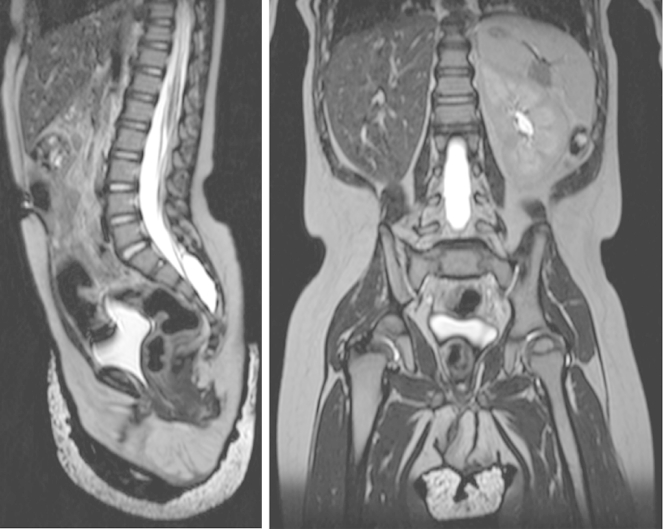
Sagittal (left) and coronal (right) T_2_-weighted magnetic resonance imaging views of the abdomen and pelvis, showing right renal agenesis and complete absence of Müllerian/paramesonephric duct structures.
